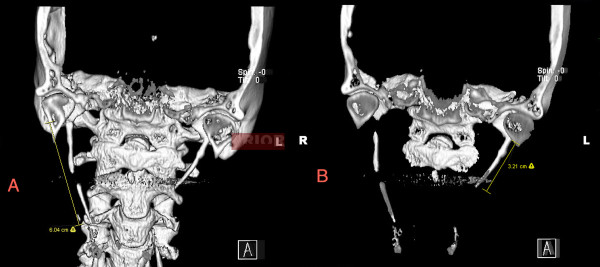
Three-dimensional reconstruction of pre-styloidectomy maxillofacial computed tomography scan showing elongated styloid processes. (A) The right styloid process is extremely elongated, at approximately 6cm. The tip of the right styloid process has been separated from the main process as a result of the 1997 manual fracture procedure. The remaining superior portion of the right styloid process is similar in length to the left styloid process. (B) The left styloid process is moderately elongated at 3.2cm.
